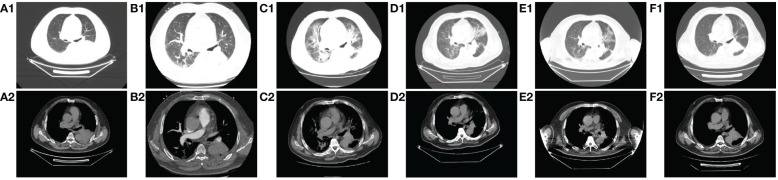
Comparison of CT images of patients before and after treatment with methylprednisolone and antibiotics. A1-A2: before immunotherapy (d-27); B1-B2: after 2 cycles of chemotherapy combined with immunotherapy (d45); C1-C2: immune pneumonitis before treatment with methylprednisolone and antibiotics (d88); D1-D2: day 4 after treatment of immune pneumonitis with methylprednisolone and antibiotics (d91); E1-E2: day 10 after treatment of immune pneumonia with methylprednisolone and antibiotics (d97); F1-F2: day 17 after treatment of immune pneumonia with methylprednisolone and antibiotics (d104).
